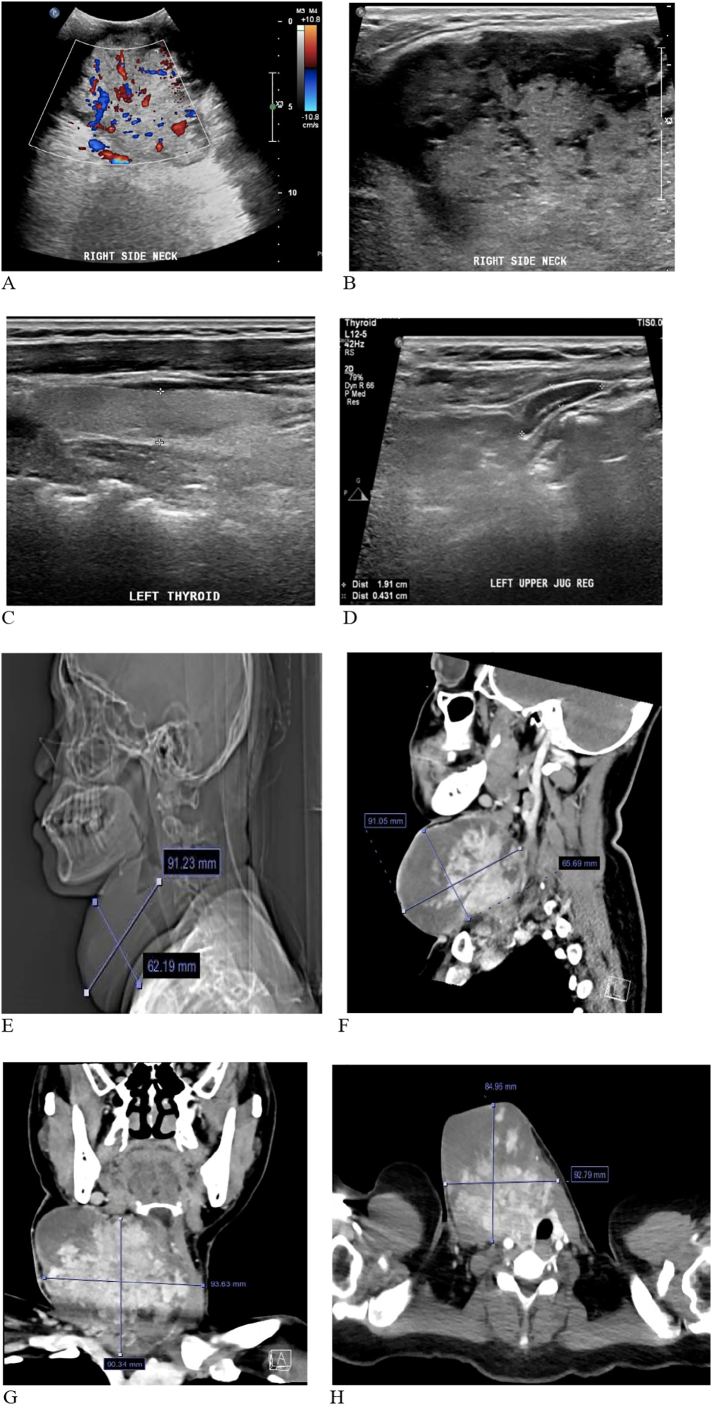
Thyroid imaging. Ultrasound. (A) Right lobe revealing large complex heterogeneous mass (9.4 × 8 cm) with midline extension from which right lobe is inseparable. (B) Doppler evaluation of right lobe showing internal vascularity within the lesion. (C) Unremarkable left lobe. (D) Largest lymph node (19 × 4 mm) in left upper jugular region. Head and chest CT findings. (E) and (F) Plain and contrast sagittal views revealing large right thyroid mass with pressure effect on adjacent structures. (G) and (C) Coronal and transverse views revealing large thyroid mass.
